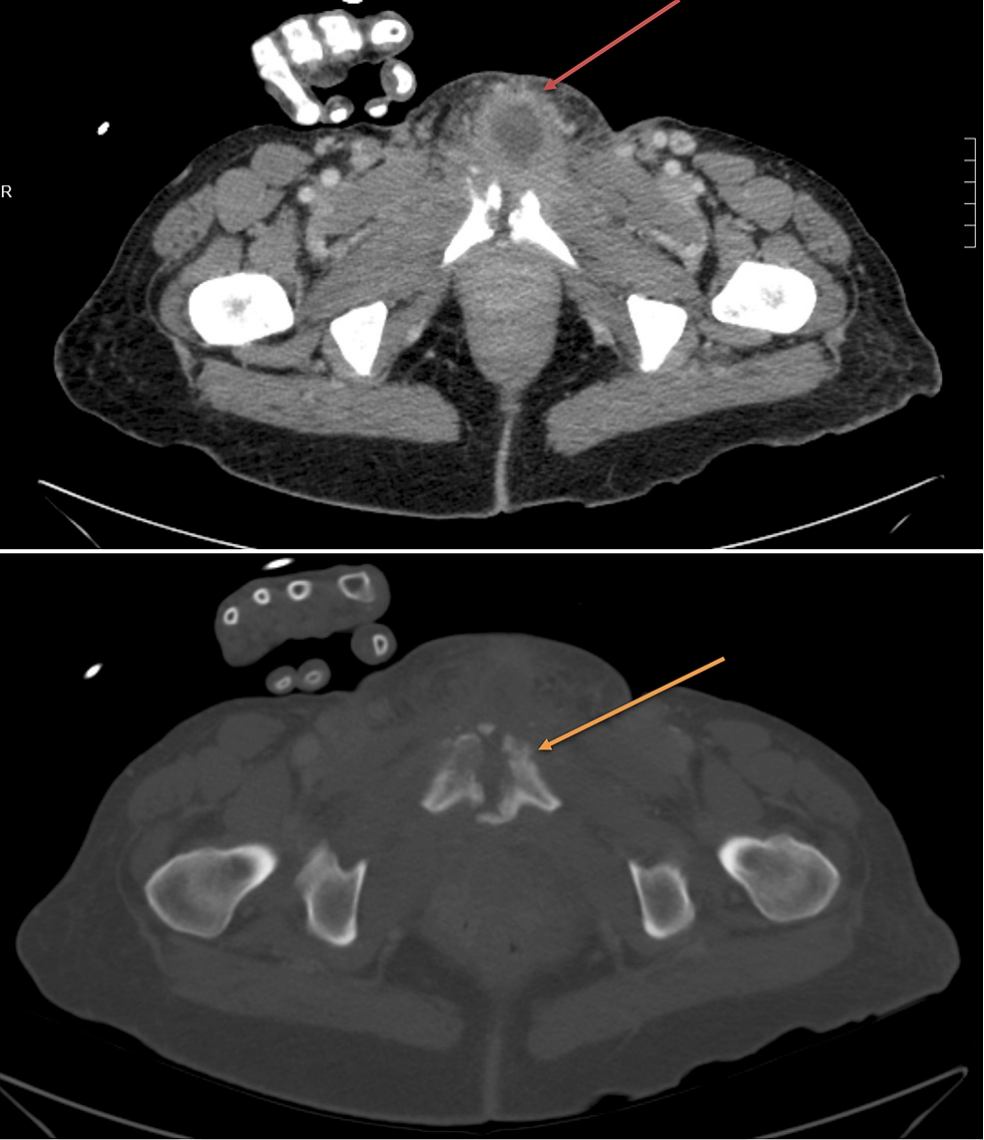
Venous-phase CT of the abdomen and pelvis. Red arrow indicates large overlying phlegmonous changes and anterior multi-loculated abscess, and orange arrow indicates destruction of the pubic symphysis with cortical erosions. Soft tissue swelling anterior and posterior to the pubic symphysis consistent with septic arthritis was also noted.
